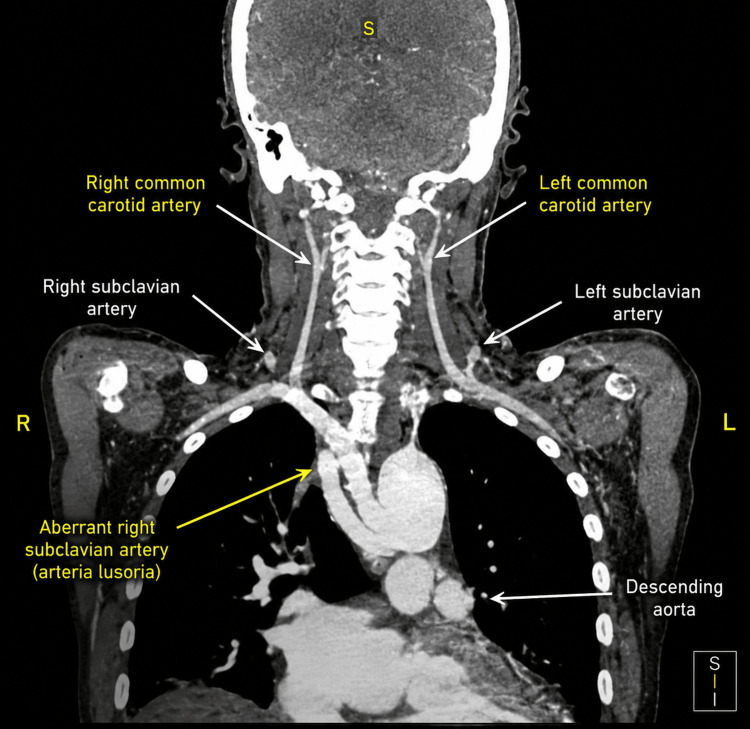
Coronal computed tomography angiography demonstrating an aberrant right subclavian artery (arteria lusoria) arising distal to the left subclavian artery.
